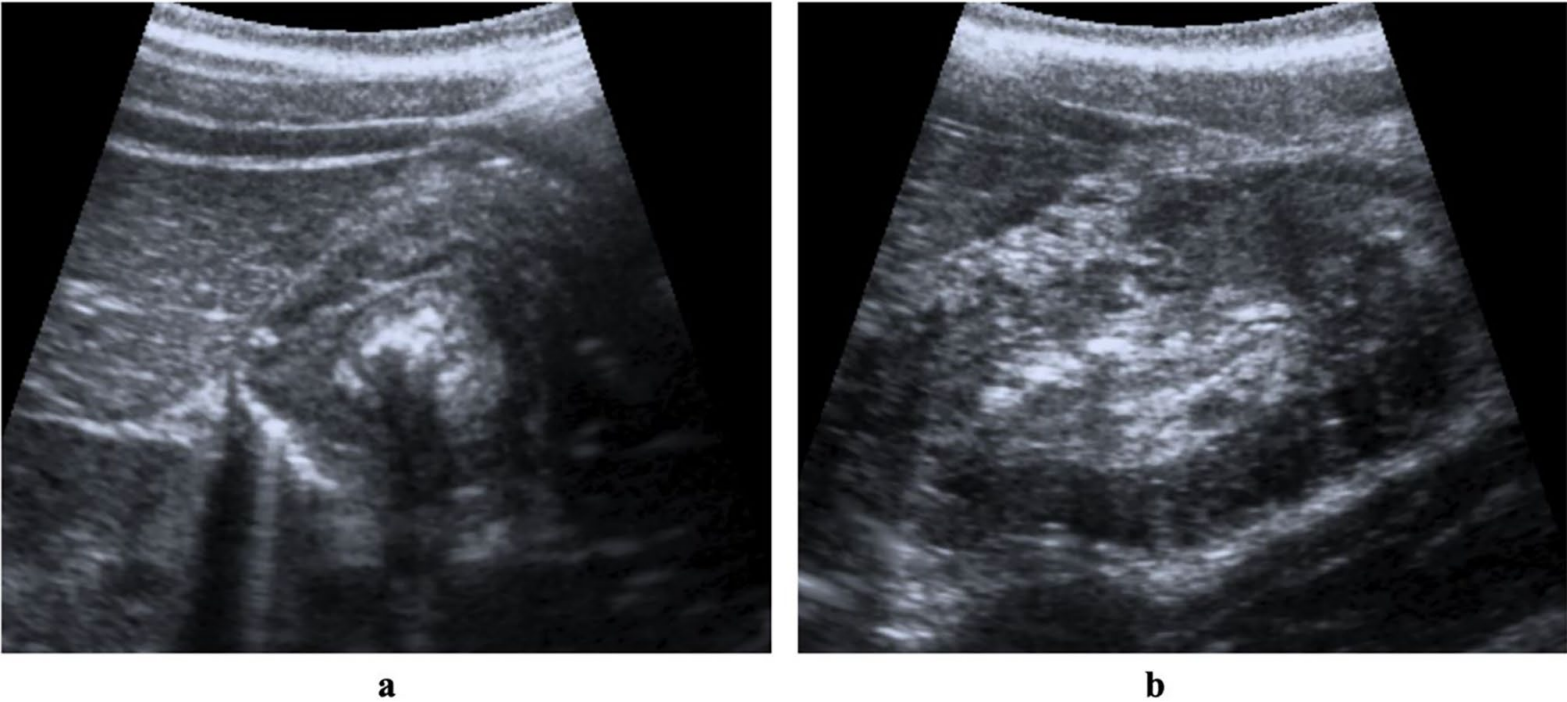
Right upper quadrant grayscale ultrasound images. a The typical bowel-within-the-bowel US appearance of ileocolic intussusception in transverse plane (doughnut or target sign). b The typical appearance in the longitudinal plane (pseudokidney sign)
